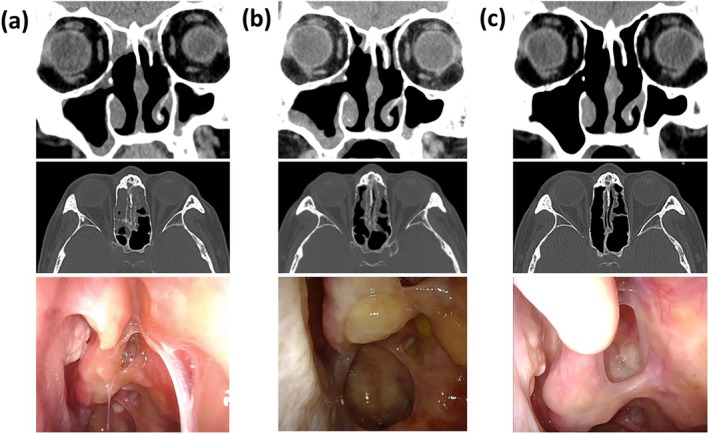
Paranasal sinus computed tomography images and endoscopic views of the left nasal cavity obtained (a) before dupilumab administration, (b) at the time of switching from dupilumab to mepolizumab and (c) 10 months after mepolizumab administration.
